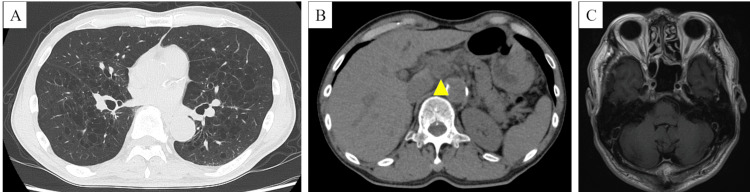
Computed tomography and gadolinium-enhanced head magnetic resonance imaging after the fourth course of carboplatin plus etoposide plus atezolizumab Computed tomography after the fourth course of carboplatin plus etoposide plus atezolizumab shows a decrease in the size of the tumor in the right lower lobe (A) and intraabdominal lymph node metastasis (yellow arrowhead) (B). Gadolinium-enhanced head magnetic resonance imaging shows the absence of left cerebellar metastasis (C).
